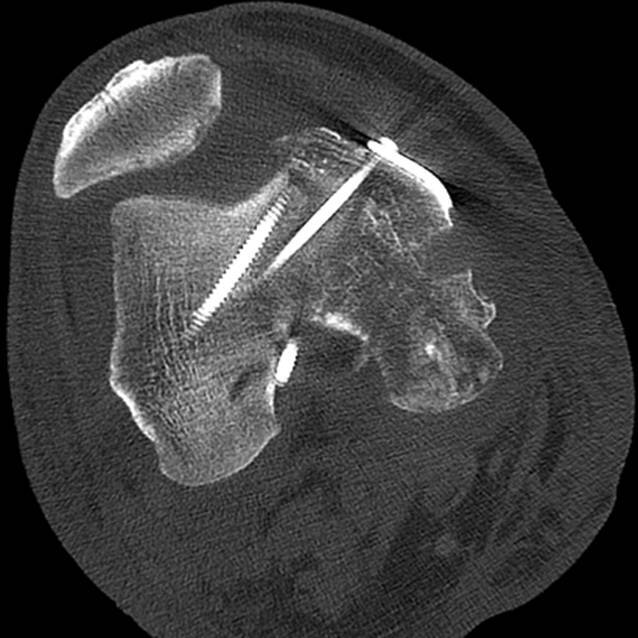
Fünf Monate nach der Rekonstruktion war der Patient ohne Hilfsmittel mobil. Im CT zeigte sich ein zufriedenstellendes Ergebnis ohne Hinweis auf einen Untergang der Transplantate (Abb. 6a, b). Allerdings waren im Bereich der chondralen Übergänge kleine umschriebene Osteolysen (Abb. 6c) zu sehen. Insgesamt sind außerdem radiologische Zeichen einer Inaktivitätsosteopenie, im Sinne fleckiger Entkalkung, abzugrenzen. Dennoch hatte der Patient keine Schmerzen im rechten Knie, konnte Spaziergänge von 2 h problemlos bewältigen und verneinte eine regelmäßige Analgetikaeinnahme. Er beklagte lediglich noch eine Flexionseinschränkung von etwa 30°. Auch die weichteilige Rekonstruktion der plastischen Chirurgen heilte bis zu diesem Zeitpunkt regulär (Abb. 6d, e).
